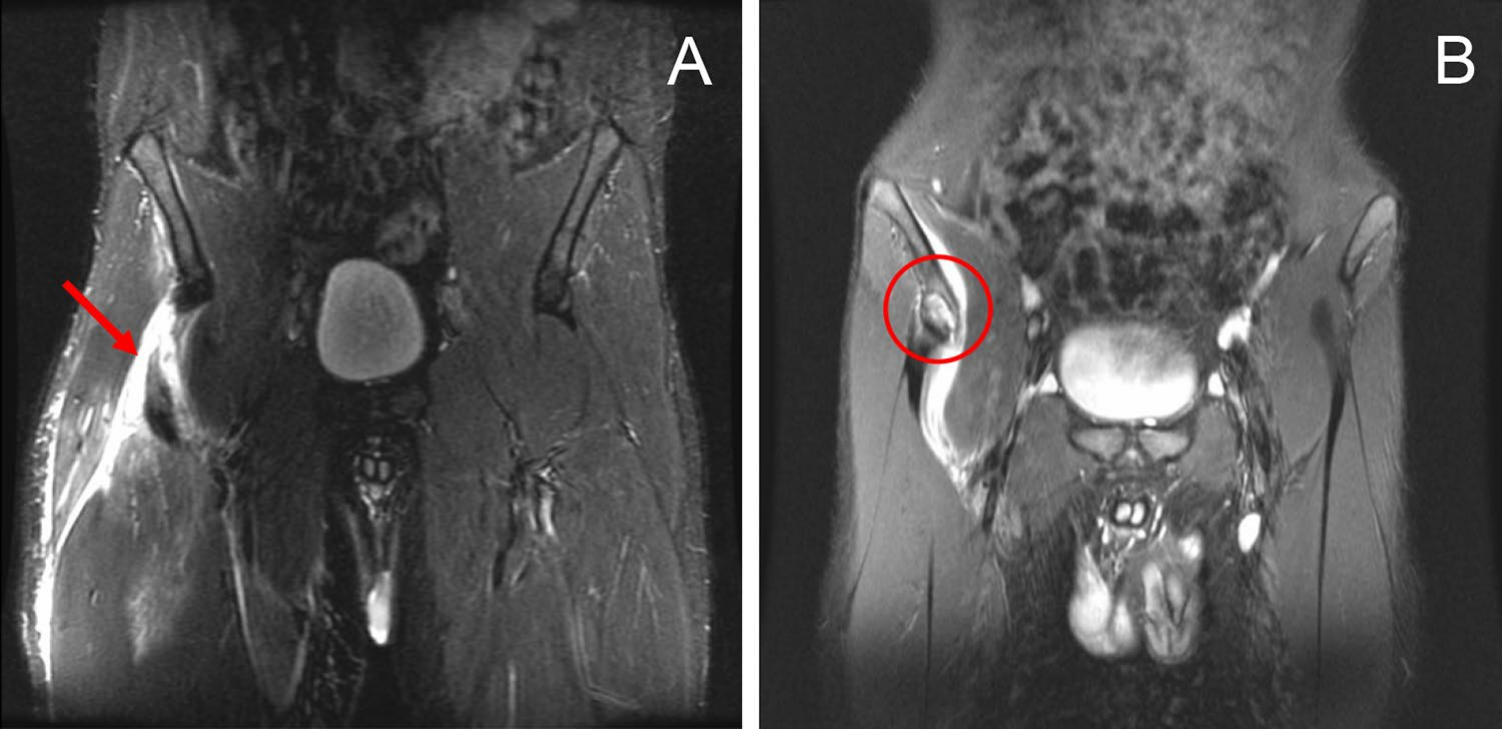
T2-weighed coronary MRI showing proximal avulsion of the rectus femoris which may appear tendinous (A) or bony (B)
